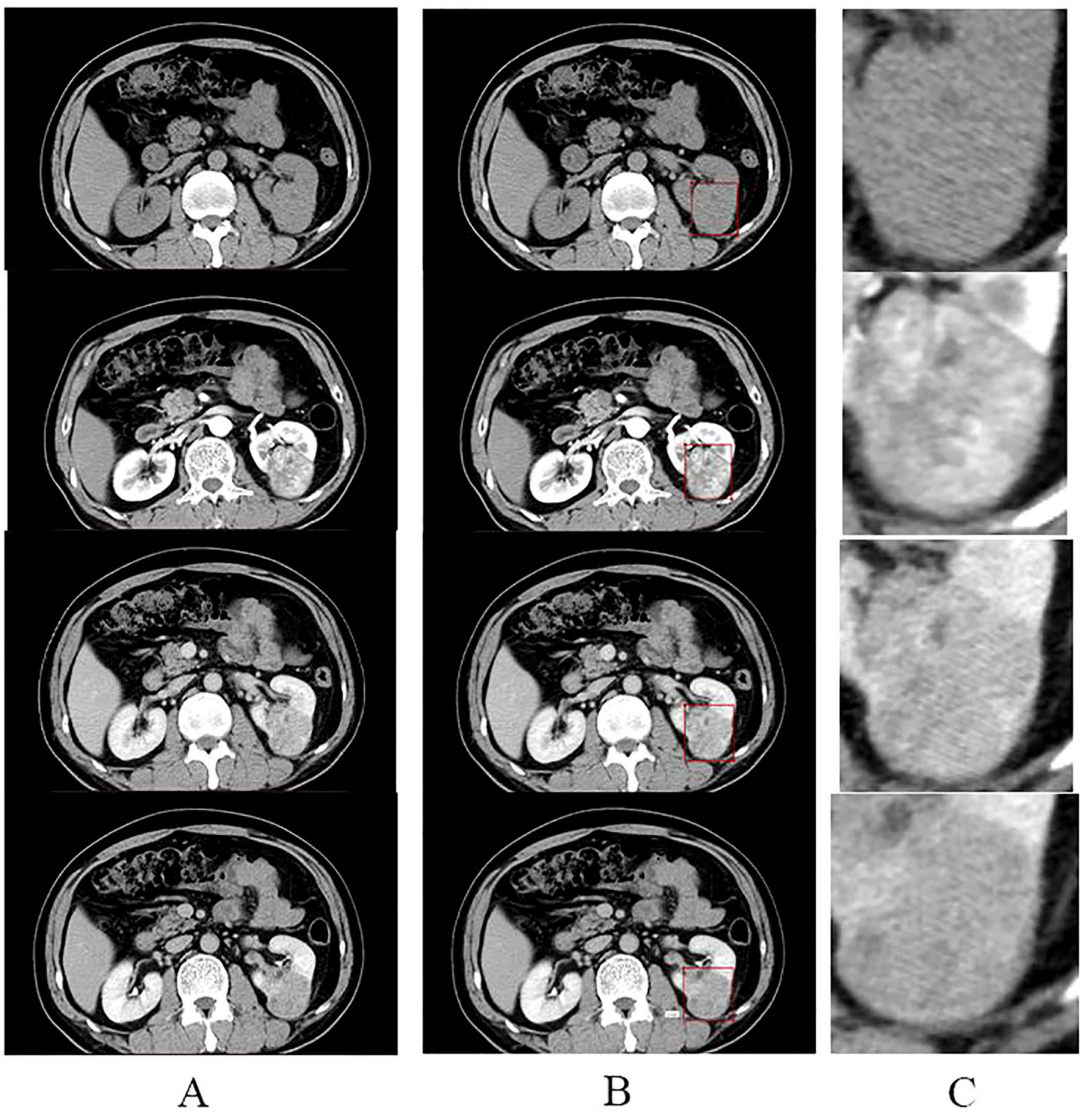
Annotation and cropping of four-phase CT images. (A) shows the four-phase CT images of the plain, corticomedullary, parenchymal, and excretory phases, from top to bottom, respectively. (B) shows the corresponding four-stage CT images that have been annotated in the rectangular box. (C) shows images in the center of (C) where the tumor area annotated in the rectangular box of (B) was cropped to remove the background and enlarged.
